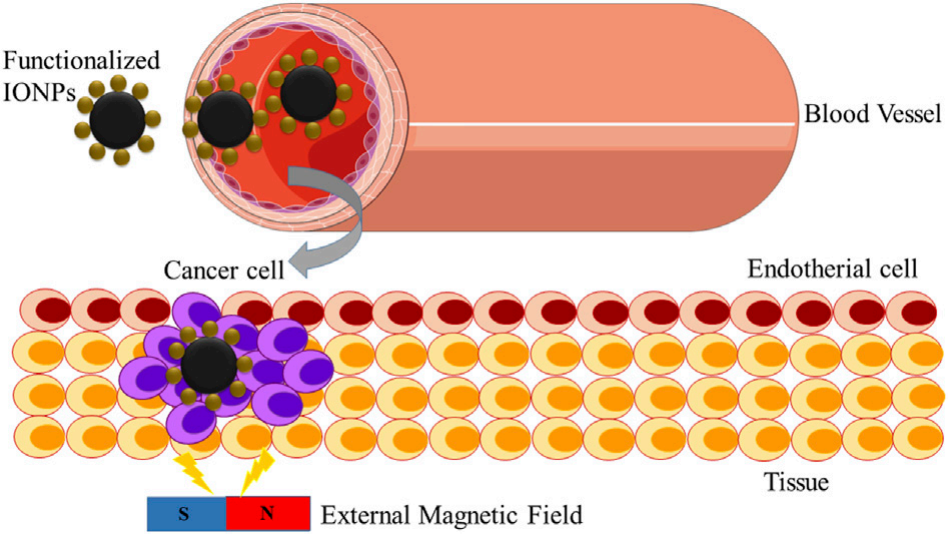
The basic steps of IONP-mediated MRI.
